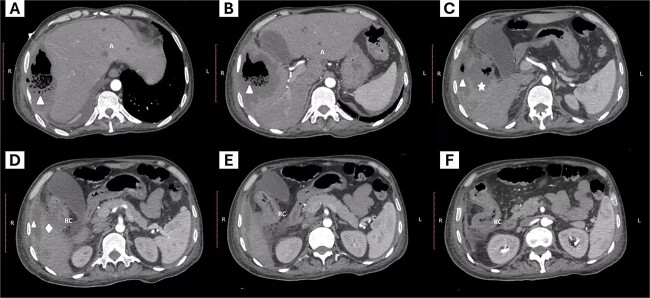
Abdominal computed tomography. The images from left to right show the cephalocaudal sequence of the tomography. (A–C) show a subphrenic abscess (triangle symbol) communicating with a hepatic abscess with compromise of the hepatic parenchyma (star symbol), and the site of colon rupture with communication to the abscess (rhombus symbol). Additionally, peripheral hepatic abscesses are visible (letter A). Images D–E show the site of communication of the colon with the abscessed region and the inflammatory process of the right colon (letters RC).
